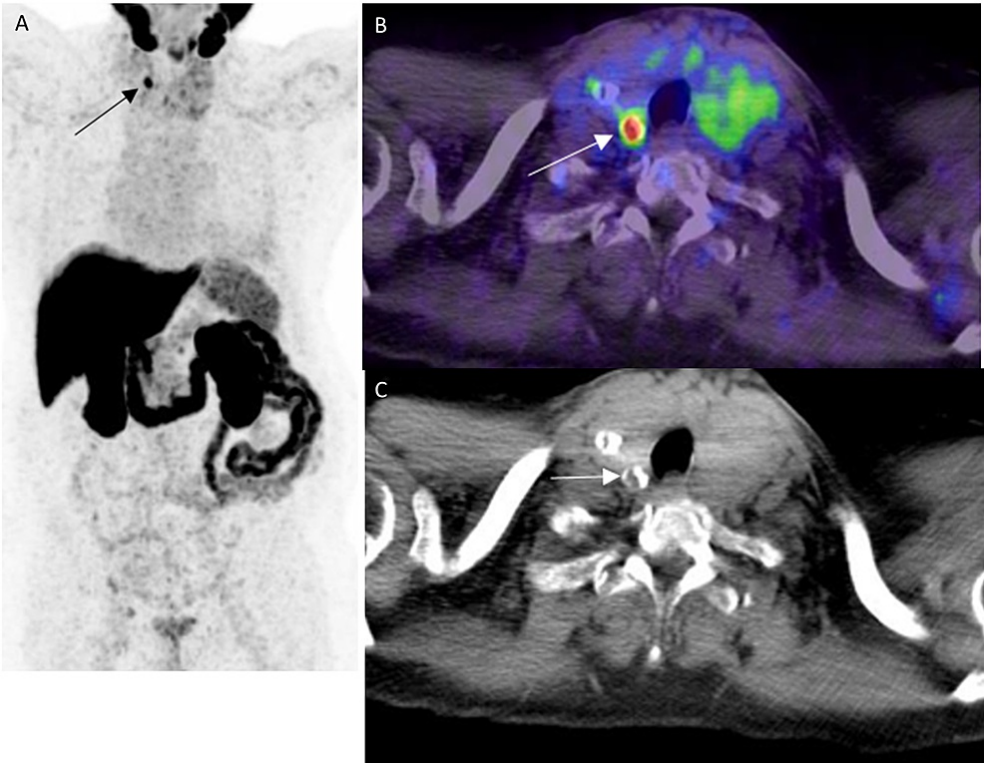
PSMA uptake in thyroid carcinoma A: 68Ga PSMA PET MIP image shows focal uptake in the right lobe of the thyroid (black arrow). B: Axial PSMA PET/CT image through the neck in the same patient shows focal intense uptake in a calcified right thyroid nodule (white arrow). FNA done showed malignant cells. C: Axial CT image through the neck in the same patient shows correlate calcified right thyroid nodule (white arrow).
